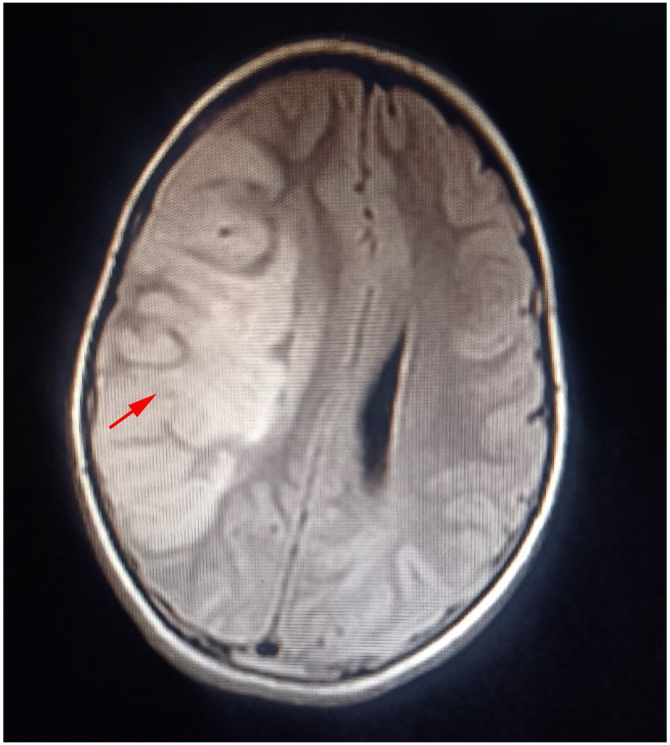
Subsequent brain MRI confirmed the presence of an ischemic stroke in the right middle cerebral artery territory due to.
